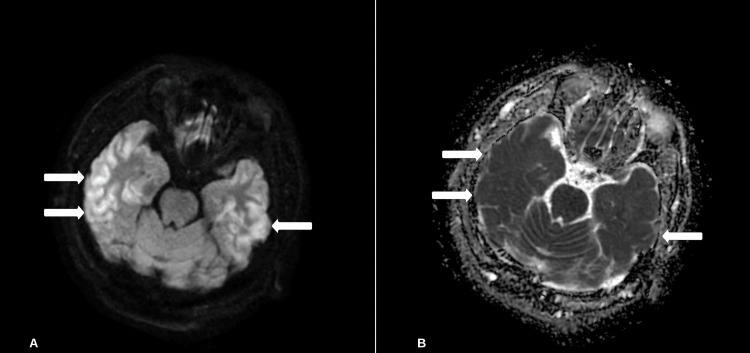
MRI of the brain axial view of DWI and ADC (A) Axial DWI sequences reveal significant bilateral temporal lobe diffusion restriction in a cortical pattern. (B) Correlating ADC sequence. DWI: diffusion-weighted imaging; ADC: apparent diffusion coefficient.
